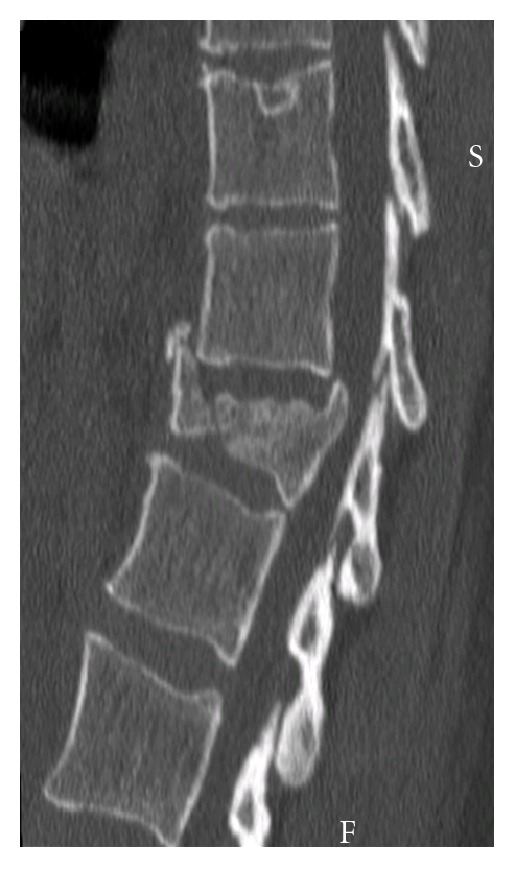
CT Scan confirming the burst fracture of T12.
